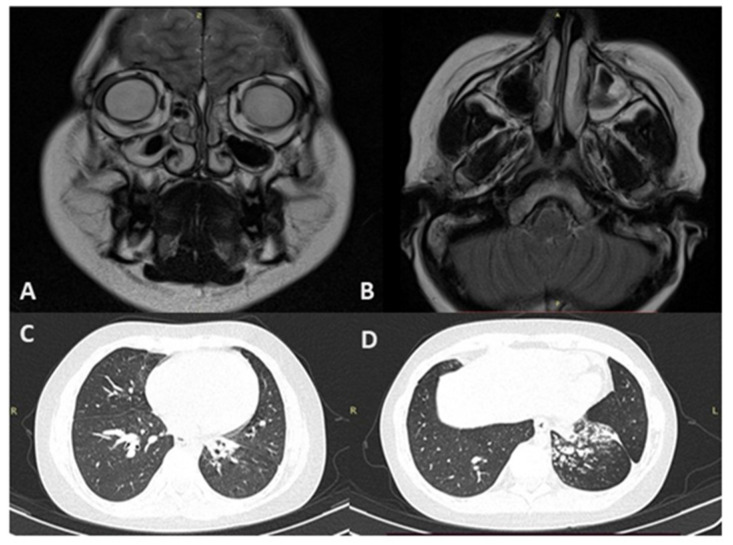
MRI of the paranasal sinuses (A,B) and CT scans of chest organs (C,D).
